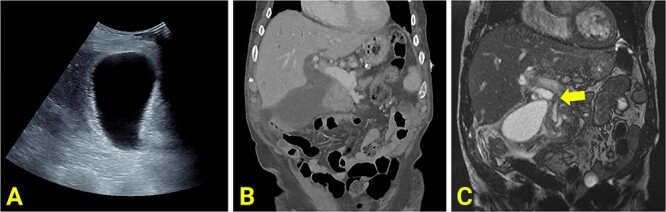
(A) Ultrasound scan showing a distended and enlarged gallbladder with a thickened wall suggestive of acute cholecystitis. No obvious gallstones are present. (B) Reconstructed contrast-enhanced coronal CT shows an enlarged and distended gallbladder, a thickened gallbladder wall, pericholecystic fluid, and a dilated common hepatic duct. (C) MRCP showing an obstructed gallstone at the confluence of the common hepatic duct, common bile duct and cystic duct (marked with arrow).
